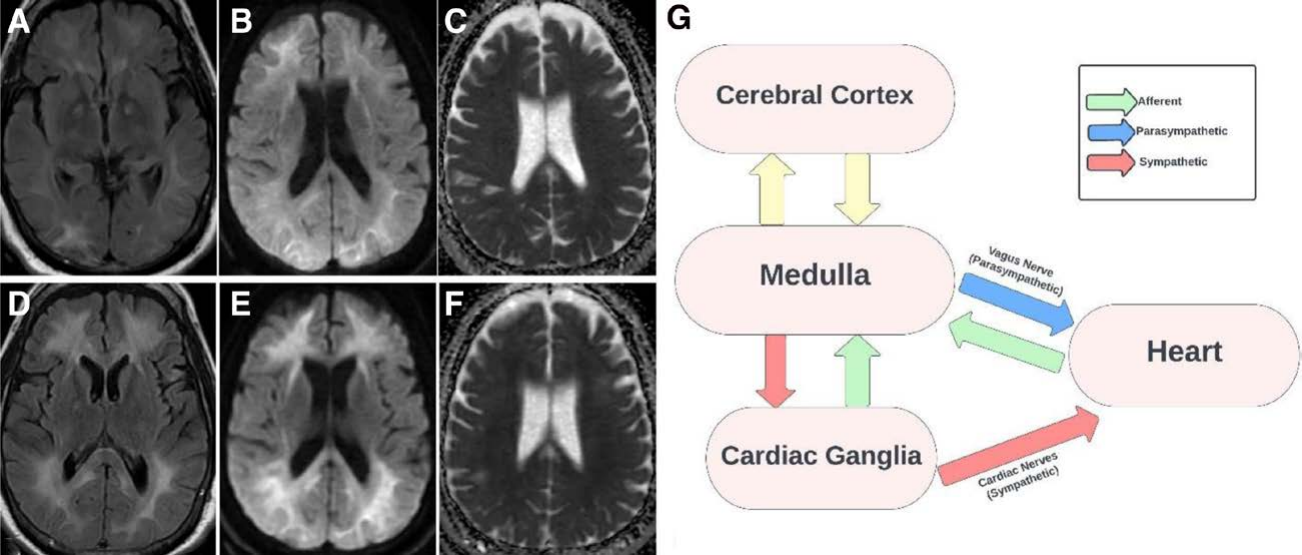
(A) MRI T2/FLAIR (B) diffusion weighted imaging (DWI) sequence and (C) apparent diffusion coefficient (ADC) on initial presentation demonstrating diffuse bilateral cortical and subcortical lesions to the white matter tracts. (D–F) Interval MRI demonstrating worsening hyperintensities in previously identified areas consistent with evolution white matter tract lesions. (G) Brain-heart communication pathways hypothesized to constitute autonomic nervous system homeostasis and heart rate variability. MRI = magnetic resonance imaging.
